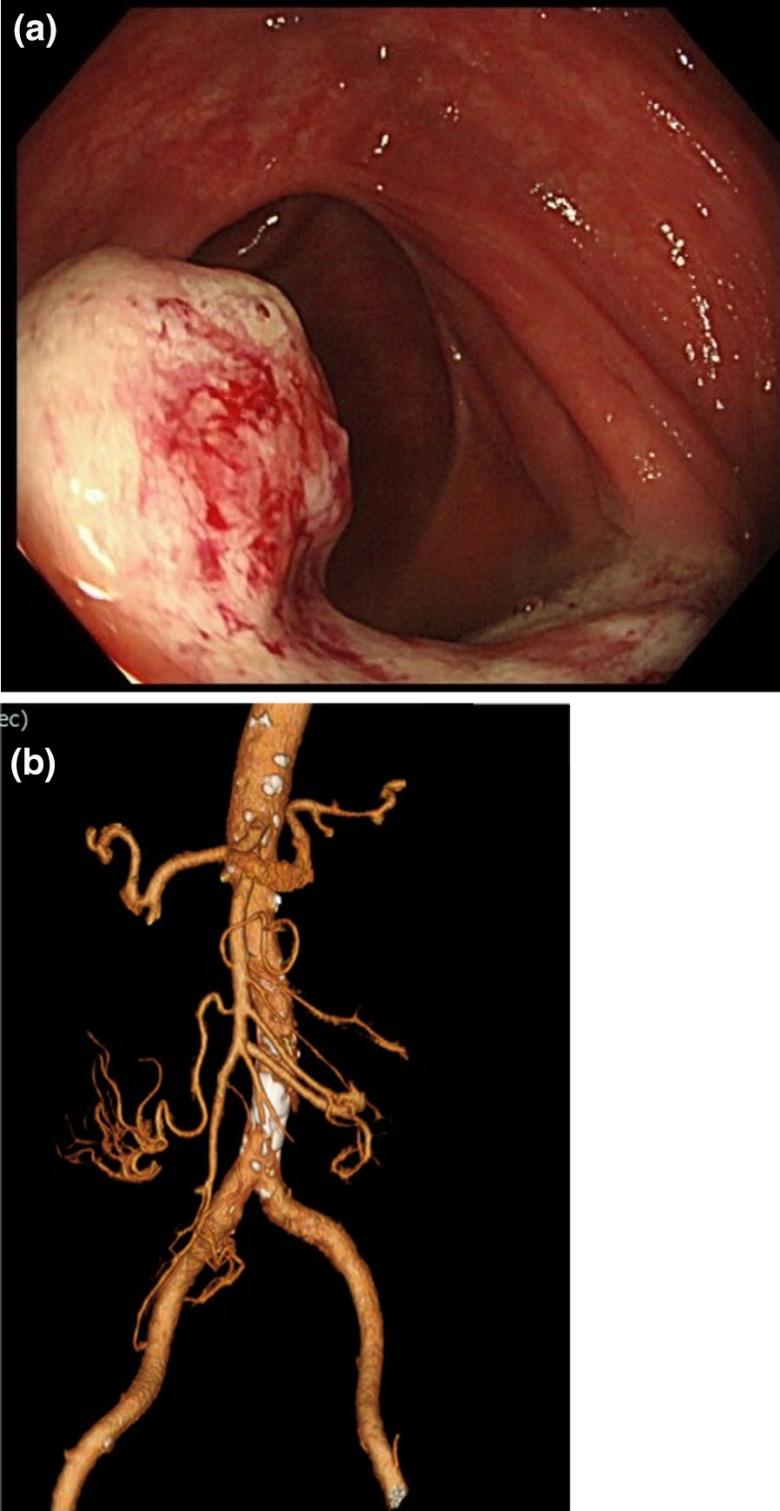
a Colonoscopy findings. A mossy-white, elevated lesion was found at the anastomosis of the ileum-transverse colon. b Preoperative 3D-CT angiography showed that the root of the middle colonic artery was preserved, which is responsible for blood flow on the anal side of the anastomosis
